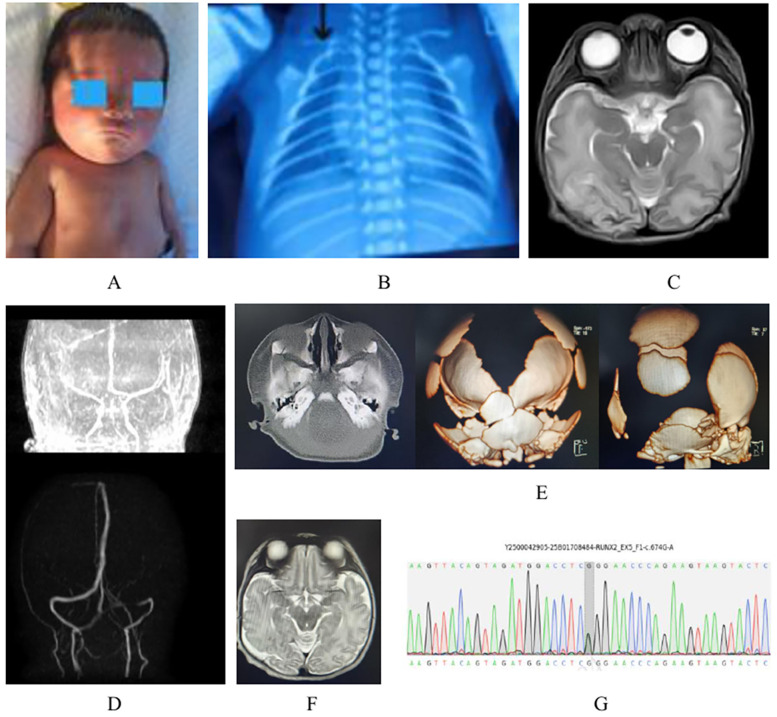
(A) The patient at his age 7 days. (B) Initial chest x-ray obtained on admission. (C) Brain MRI findings at 1 week of life: Cerebral infarction and hemorrhagic (D) Cerebral arterial (MRA) and venous (MRV) imaging at 1 week of life. (E) Nasal bone CT and cranial CT reconstruction. (F) Follow-up brain MRI at 42 days of life. (G) Genetic testing results of the proband (RUNX2 variant).
